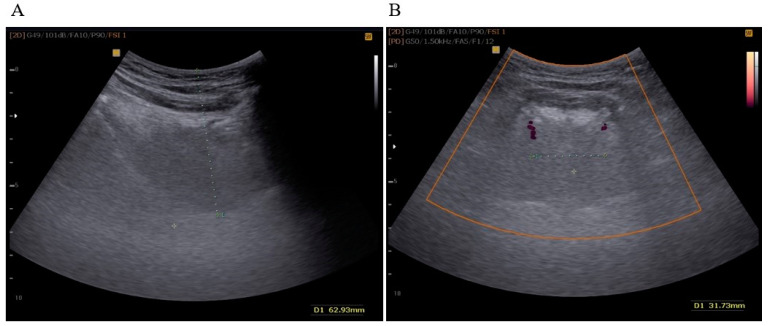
(A) TBT measured in the sagittal plane (green dots); (B) DLA measured in the coronal plane in the PD (Power Doppler) mode (green dots).
